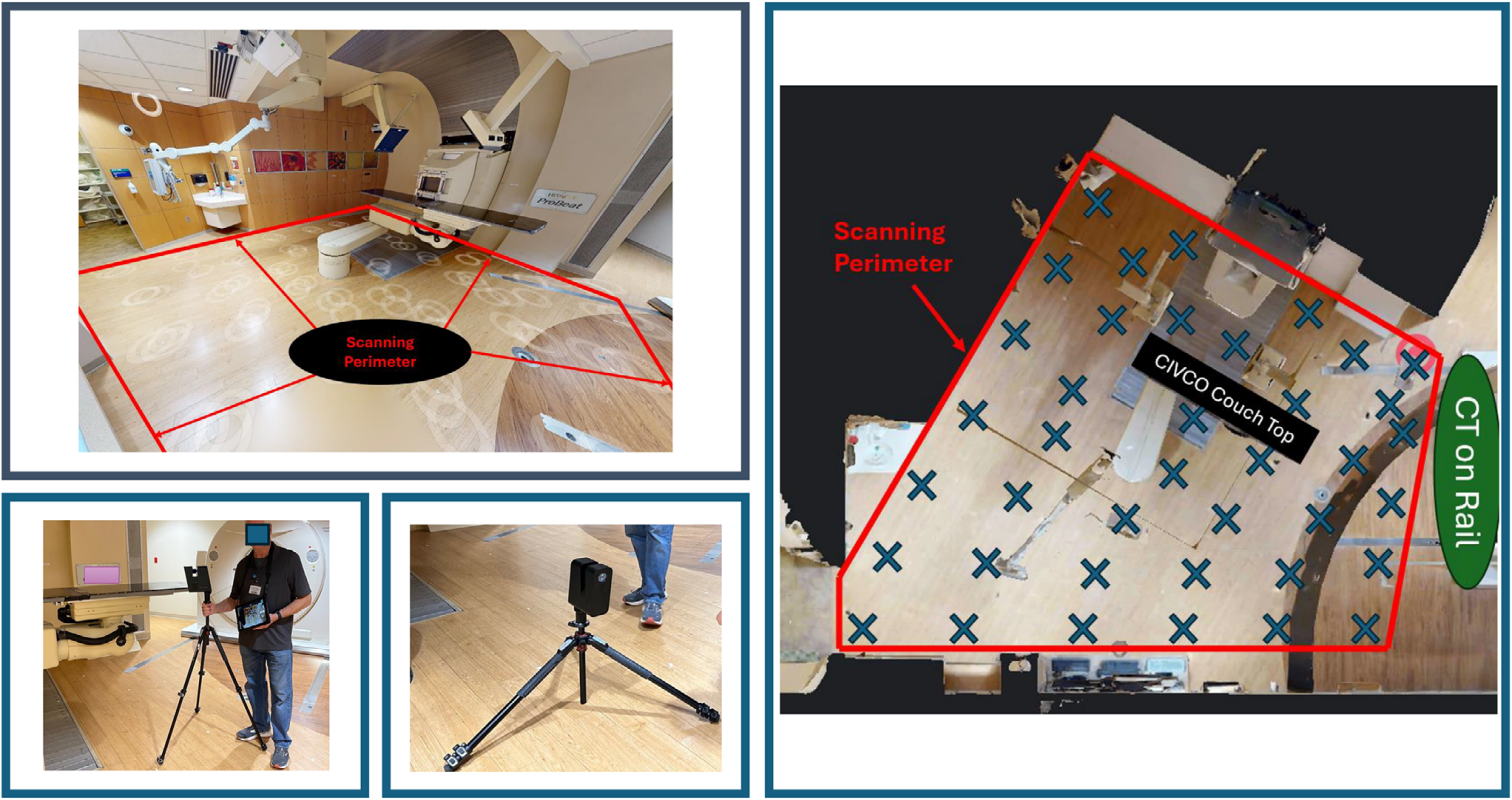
On the top left and the right of this figure, the red lines define the farthest boundaries where the Matterport camera was positioned. The “x” symbols indicate the planned camera acquisition locations. The treatment couch top is located approximately near the center of the scanning perimeter. The CT‐on‐Rail system is located outside of the scanning perimeter. The bottom of the figure shows two of the three camera acquisition heights: mid‐position and the low position.
